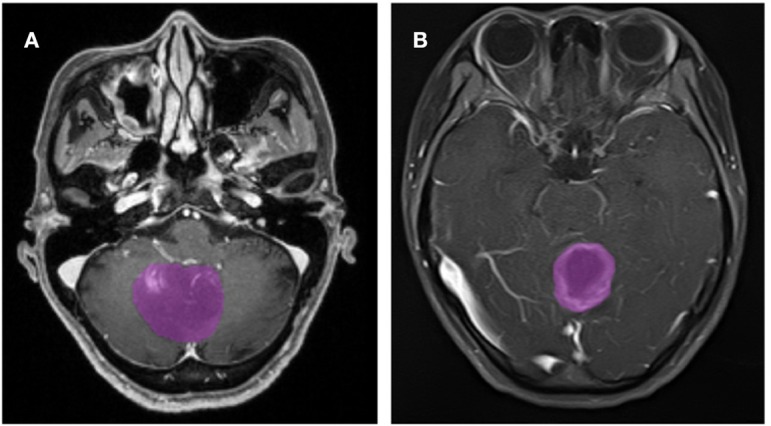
Examples of two cases from the contrast-enhanced MR images in patients with glioblastoma and ANA. (A) Contrast-enhanced images with ANA, (B) contrast-enhanced images with glioblastoma. ANA, Anaplastic astrocytoma.
